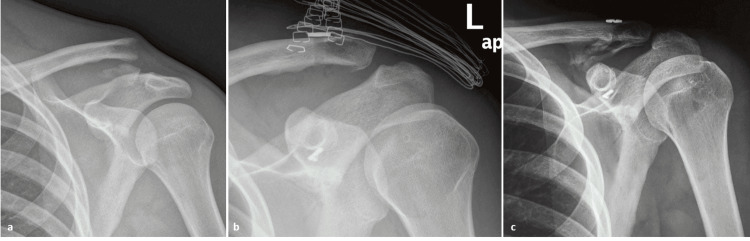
Radiological evaluation of patient 5. (a) Preoperative Zanca radiograph of an extralateral distal clavicle fracture with marked medial fragment displacement. (b) Postoperative Zanca radiograph showing excellent reduction of the fragment. (c) Final follow-up Zanca radiograph showing maintenance of reduction and calcification of coracoclavicular ligaments, a sign of adequate healing. The patient had excellent outcome scores.
